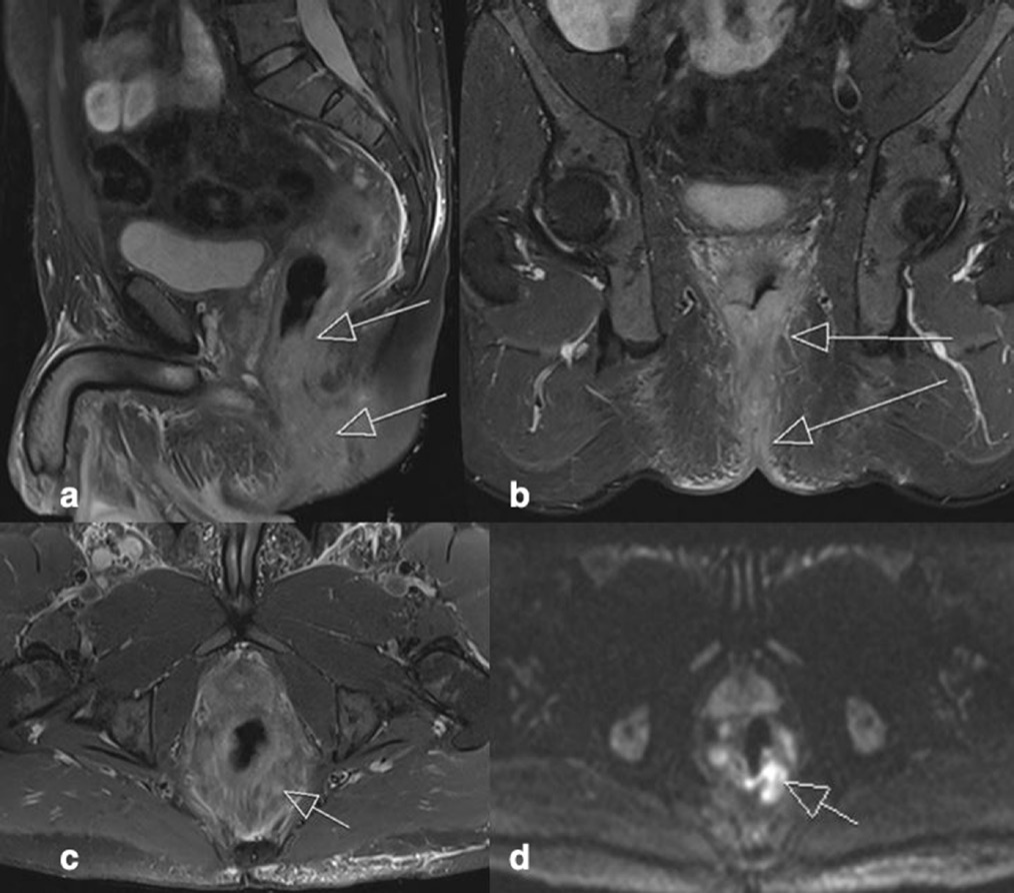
A. Sagittal STIR image demonstrates high signal of the rectum and anal canal in keeping with severe active proctitis and extensive inflammation of the anal canal (arrows). Figure 2B. Coronal STIR image demonstrates high signal extending from the anal canal to the perianal/perineal regions in keeping with extensive inflammation of perianal skin (arrows). Figure 2C. Axial STIR image demonstrates prominent mesorectal lymph nodes secondary to the adjacent proctitis. Figure 2D. Axial DWI image demonstrates corresponding restricted diffusion in the mesorectal lymph nodes.
